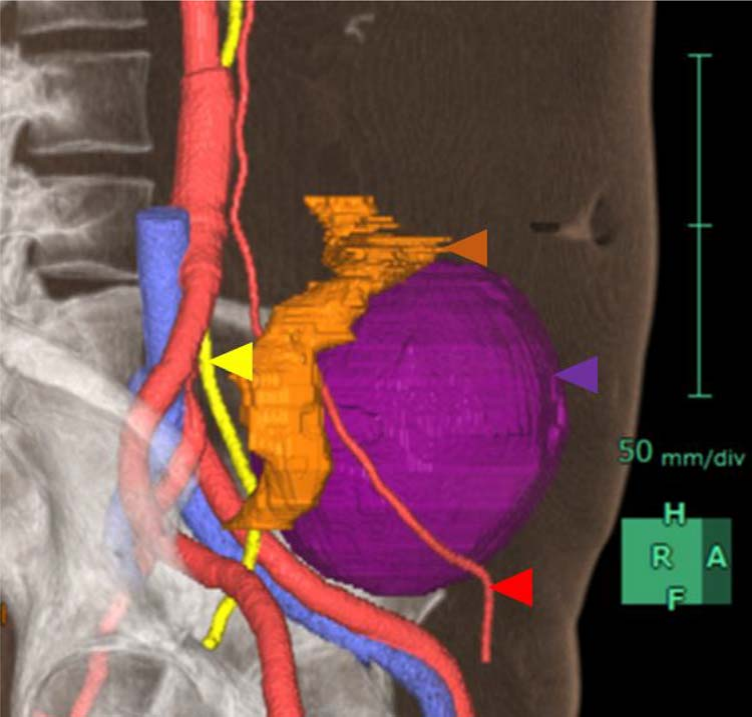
3D image constructed based on CT scans. Purple area is the tumor, yellow area is the left ureter, red areas are the left testicular arteries and orange area is the sigmoid colon.
